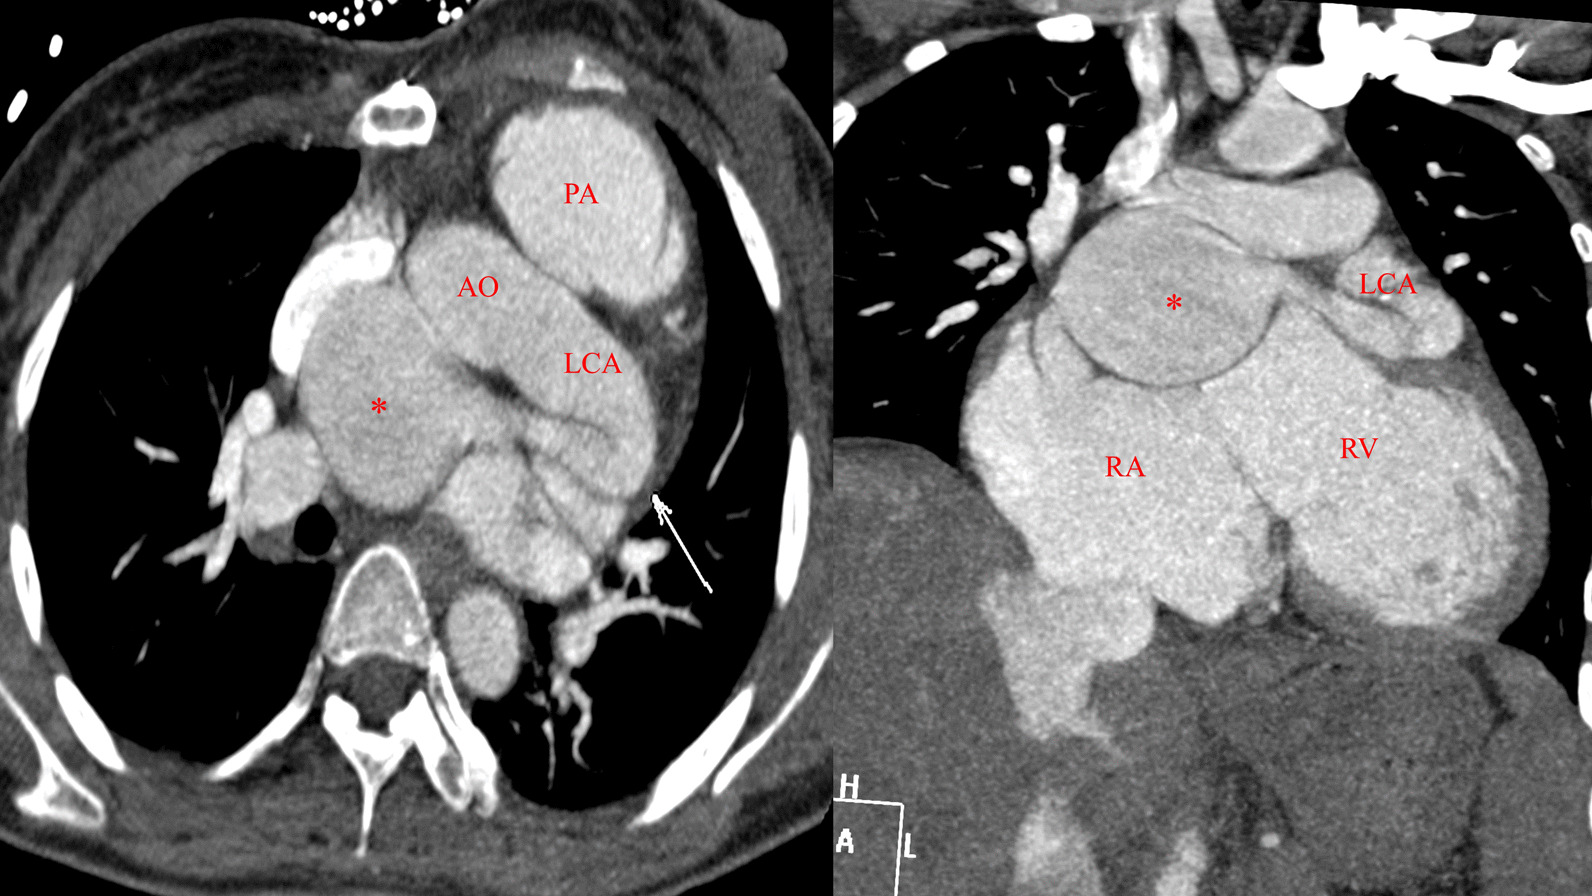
Multi-slice computed tomography angiography confirmed the diagnosis of LCA and revealed the significant enlargement of the LCA (arrow), the measured diameter of the coronary aneurysm (asterisk) was 45 × 56 mm and of the orifice of fistula was 9 mm (LCA: Left coronary artery; RV: Right ventricle; RA: Right atrium; AO: Aorta; PA: Pulmonary Artery)
